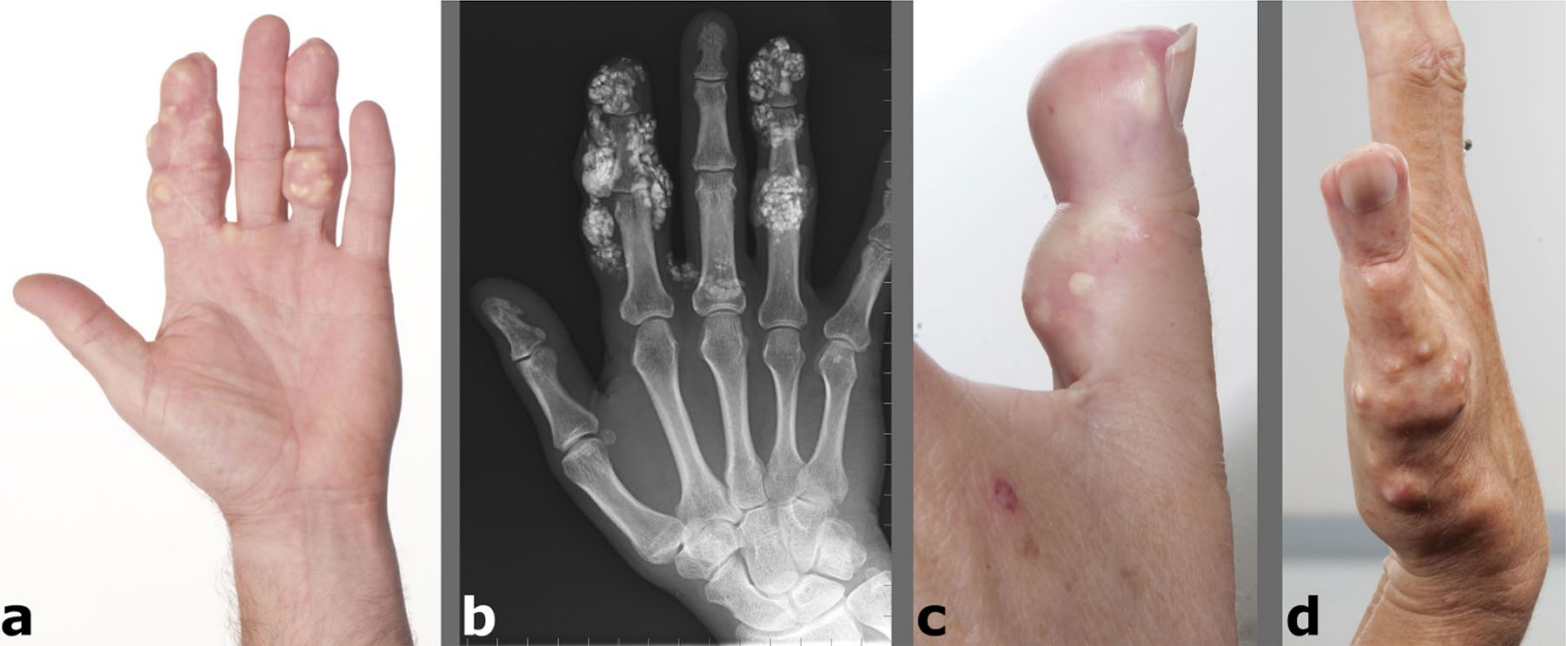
Images depicting examples of SSc-related calcinosis. *Calcinosis cutis* in the first and third digits of the hand (a,b), the calcinosis is well demonstrated on plain radiography (b), and the thumb (c,d). Images copyright of Northern Care Alliance NHS Foundation Trust.
